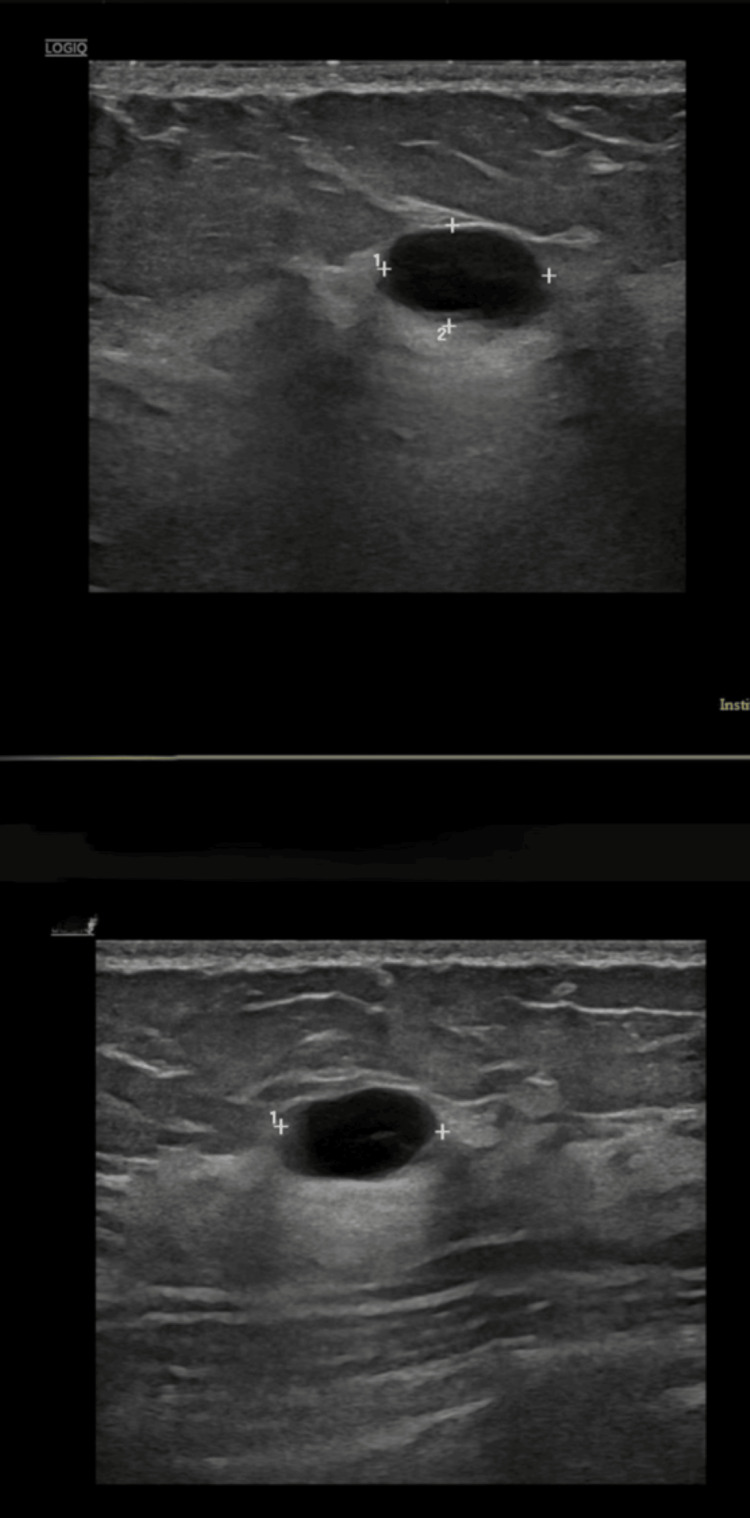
Breast ultrasound shows cystic lesions measuring 10 mm and 8.2 mm at the 2-3 o’clock position in the left breast (suggestive of simple cysts).
